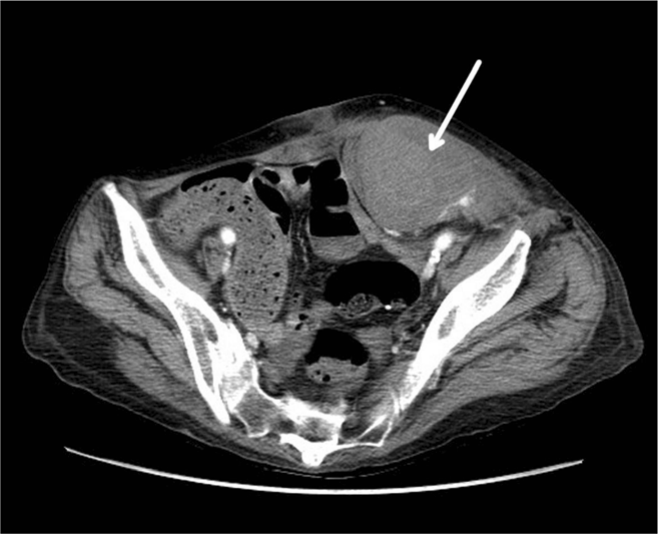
Left rectus sheath hematoma. A CT scan of the abdomen and pelvis revealing a large left rectus sheath hematoma (arrow).
